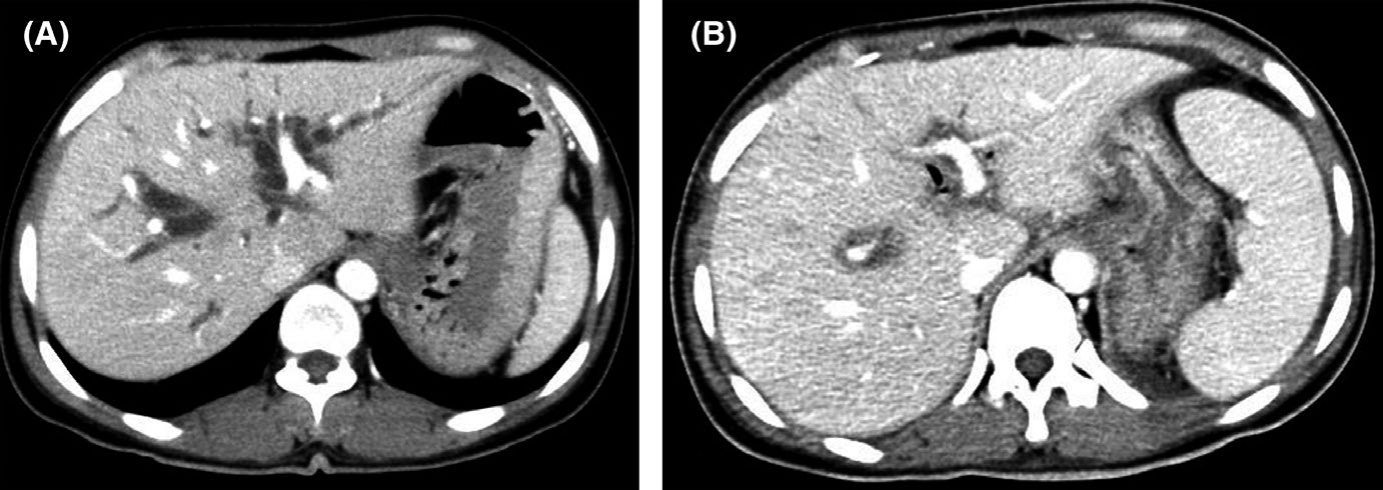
The change of splenic volume before and 6 months after operation on abdominal CT *a*. Splenic volume before operation, the maximum diameter was less than 5 rib units. *b*. Splenic volume increased significantly 6 months after operation, and the maximum diameter was more than 8 rib units
